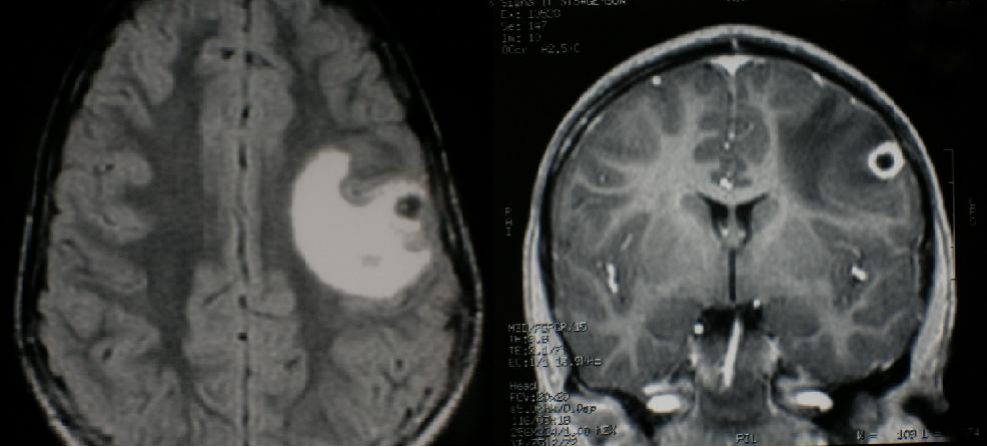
Brain MRI before specific treatment. (a) axial T2-weighted MRI shows an alteration in left frontal-parietal cortex attributing to vasogenic edema (b) coronal T1-weighted post contrast MRI shows a little ring-enhancing lesion in left frontal cortex.
